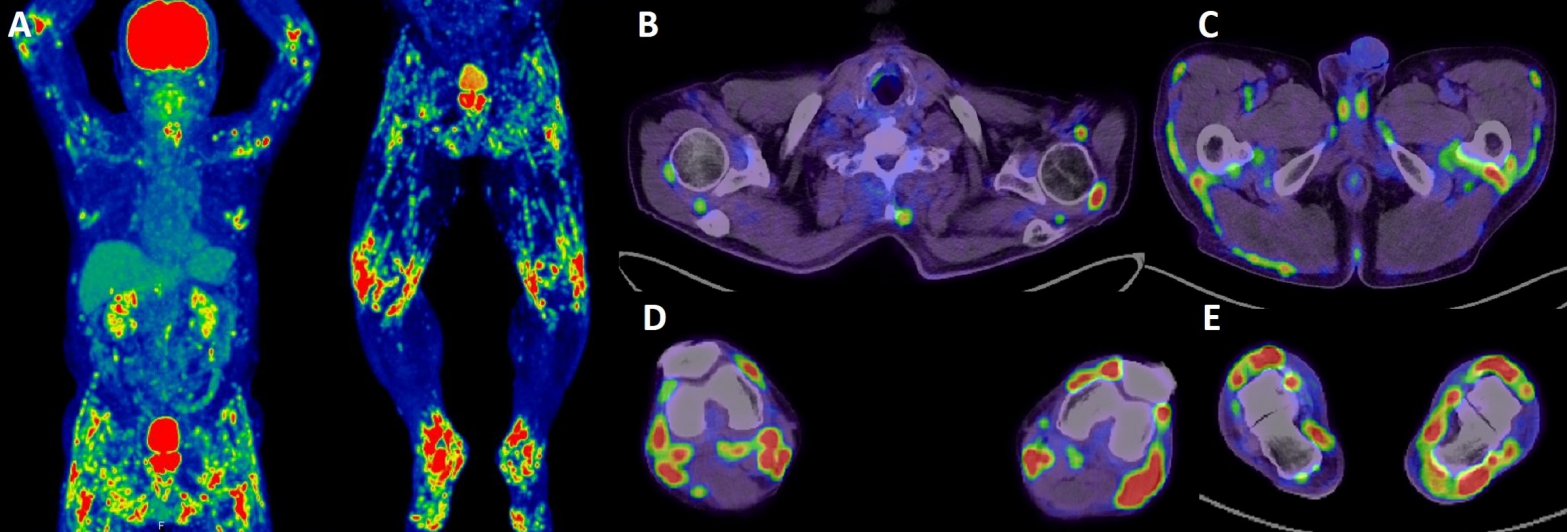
Imaging findings of the patient. This panel presents 18F-fluorodeoxyglucose positron emission tomography/computed tomography showing accumulation of fluorodeoxyglucose in numerous muscle-tendon attachment sites. (A) whole body, (B) shoulder region, (C) lumbar region, (D) knee region, and (E) foot region. SUV_mean_ ± SD of each site: attachment sites of subscapular fossa and subscapularis muscle, 3.25 ± 0.86; attachment sites of the clavicle and subclavian muscle, 2.00 ± 0.56; attachment sites of the humeral head and supraspinatus muscle, 6.85 ± 2.38; attachment sites of the humeral head and biceps brachii tendon, 4.68 ± 1.45; attachment sites of femur and gluteus minimus, 2.54 ± 0.31; attachment sites of femur and gluteus medius, 2.80 ± 0.45; attachment sites of coccyx and gluteus maximus, 5.01 ± 0.84; attachment sites of the coccyx and iliac muscle, 4.36 ± 0.71; attachment sites of the coccyx and obturatorius externus muscle, 3.46 ± 0.73; and tendon-to-bone attachment sites of knees, 12.70 ± 2.44. SD: standard deviation; SUV_mean_: mean standardized uptake value.
